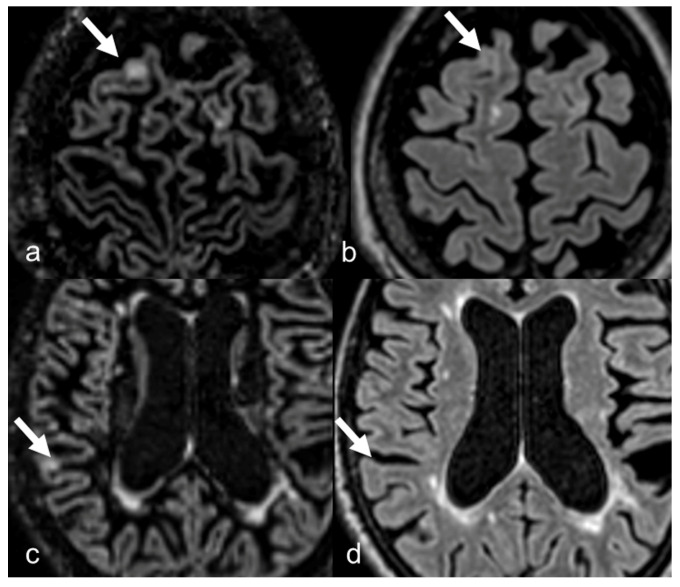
(a,c) The right cortical lesions (arrows) are well detected on DIR images; (b,d) the same lesion is unapparent on FLAIR images.
